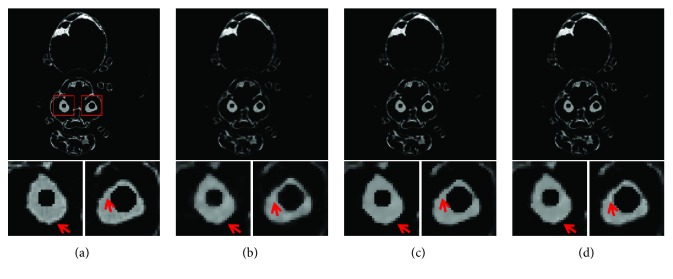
CT images of the modified bee reconstructed from consistent projections: (a) the reference image; (b) SART; (c) TV; (d) TF-L0. The second row images are the close-ups of ROIs. The places pointed out by red arrows are examples where TV and TF-L0 acquire different results.
